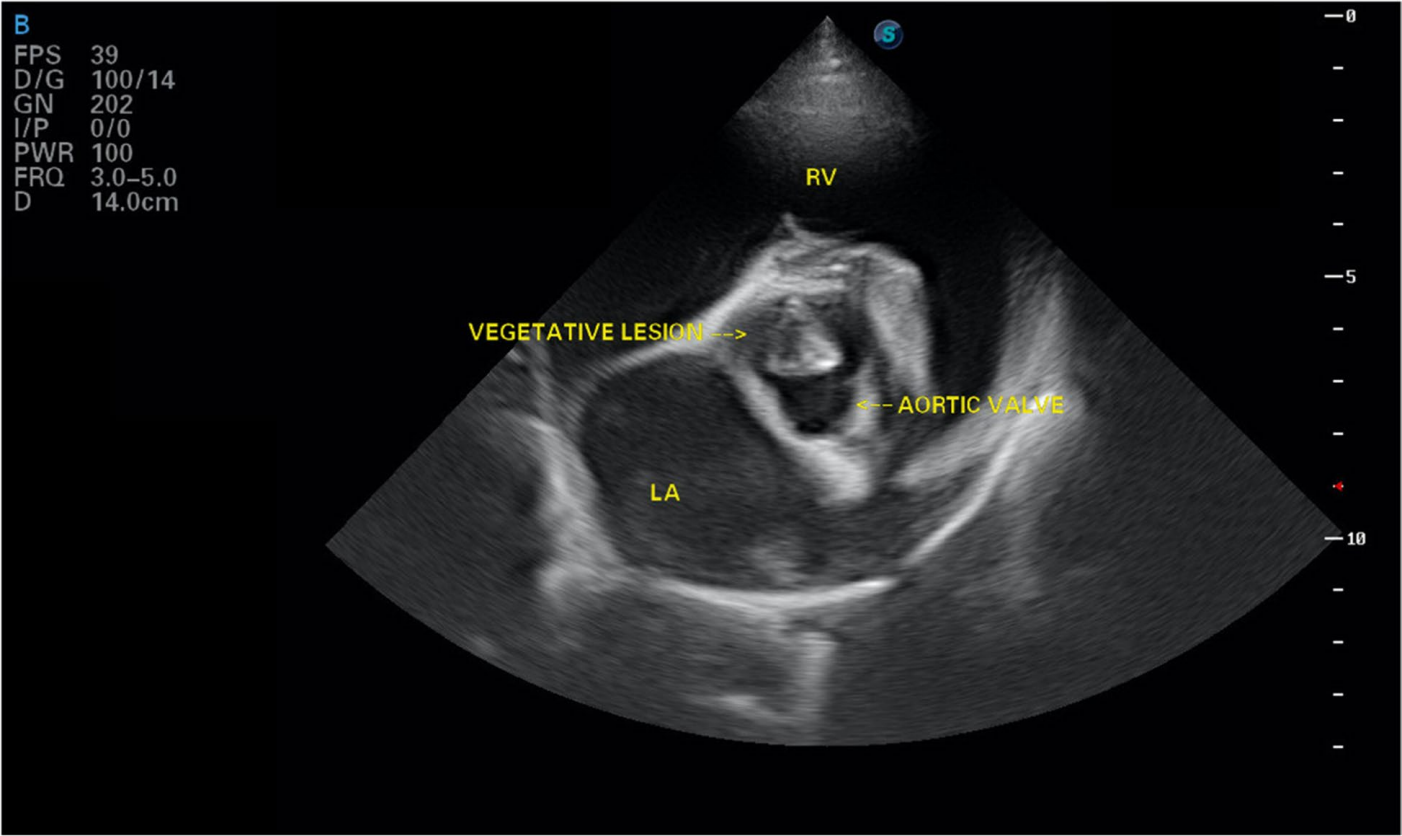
Aortic valve vegetation on short axis echocardiographic view (LA: left atrium, RV: left ventricle)
